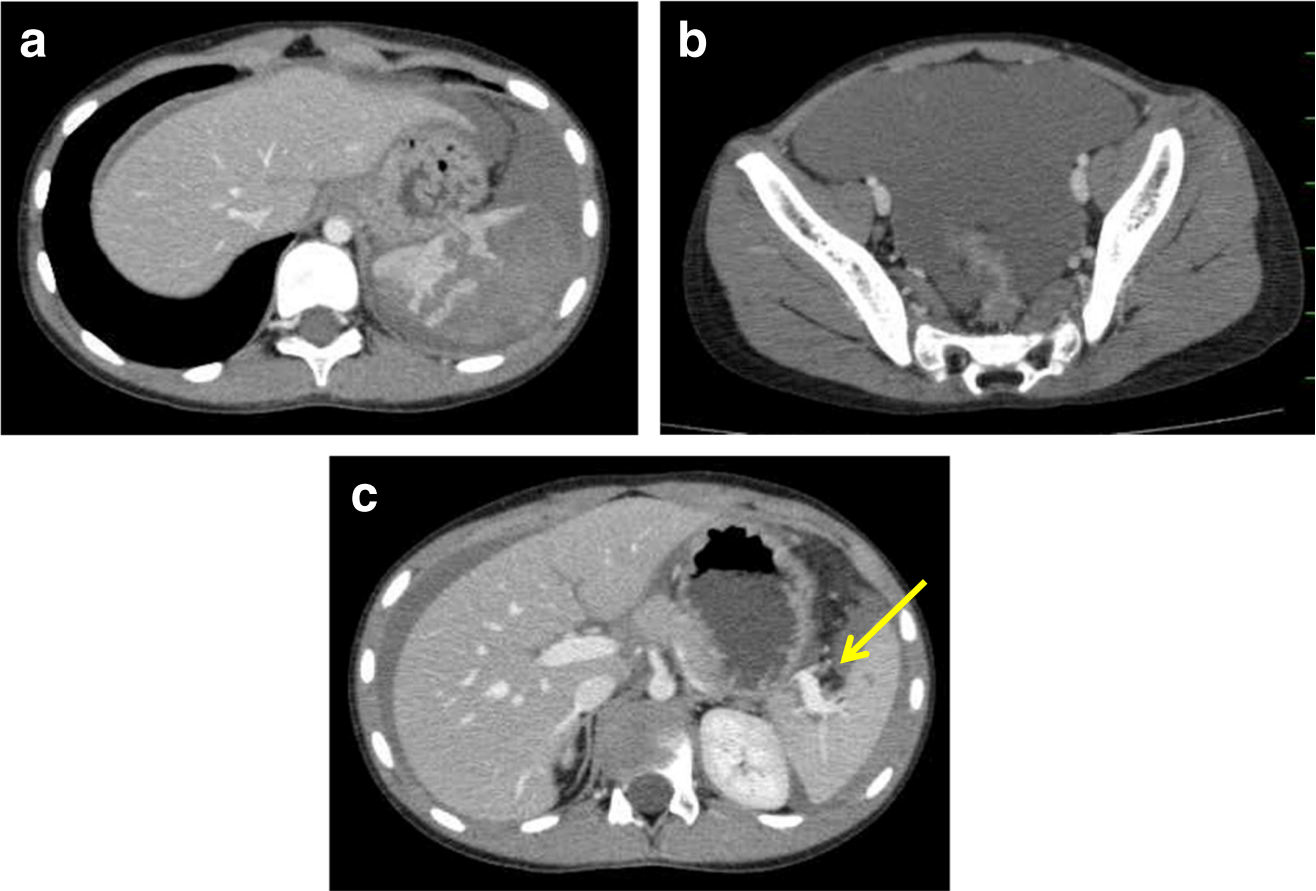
Contrast-enhanced CT at admission (21 h after injury). a Severe intraparenchymal hematoma and multiple lacerations of the spleen were noted. b. A large amount of hemoperitoneum was detected in the pelvic cavity. c The hilar vessels of the spleen were not injured, and no active bleeding was seen in the spleen (→). The injury was diagnosed as AAST grade III
